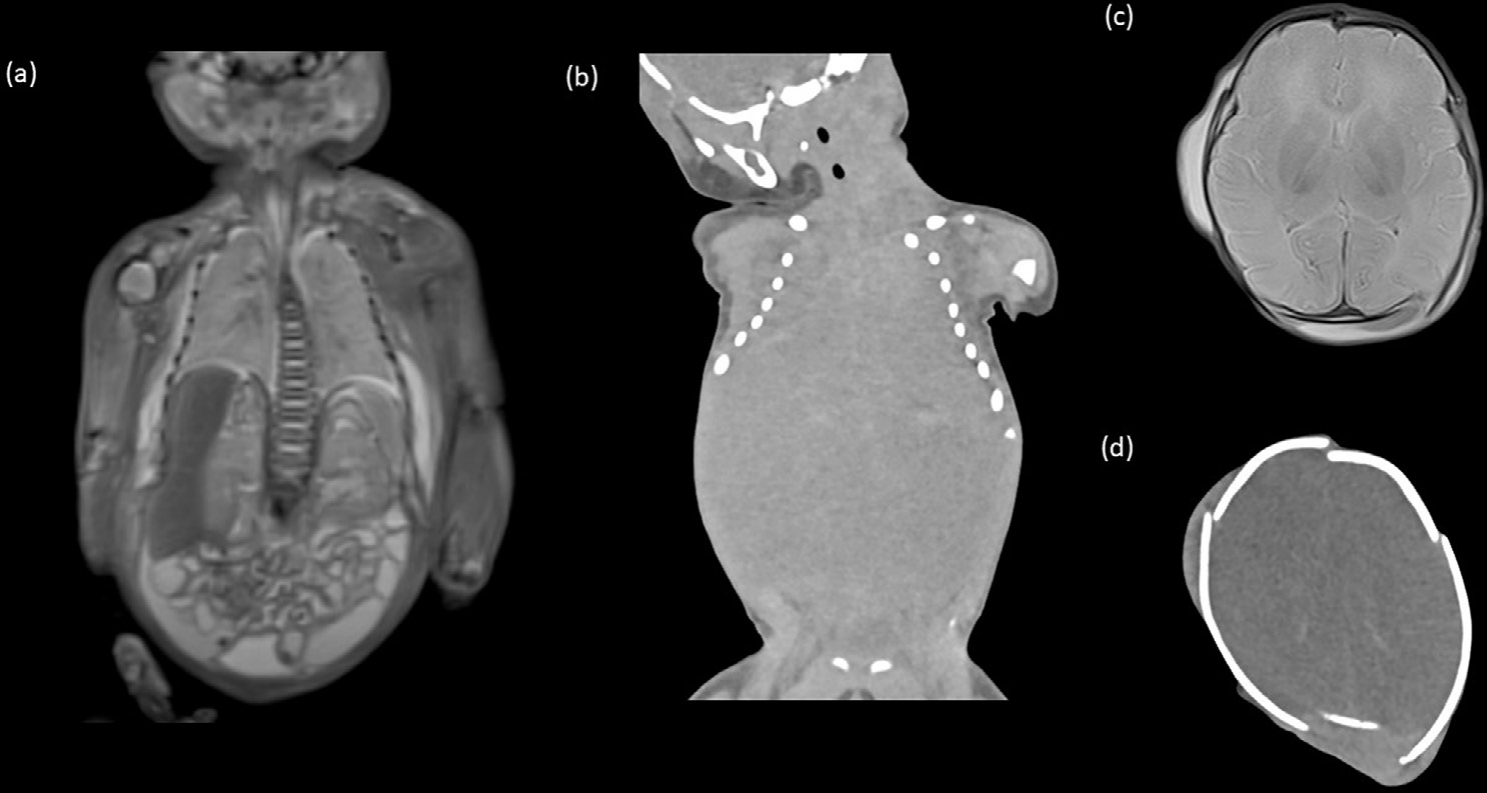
Comparison of post-mortem MRI and CT imaging in the same stillborn foetus of 36 weeks gestational age. Coronal post-mortem MRI (a) and CT (b) of the thorax and abdomen, with axial *T*
